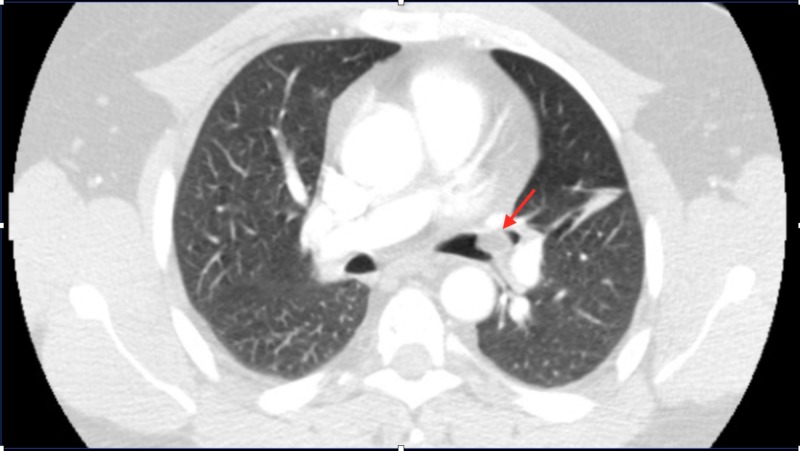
Computed tomography of the chest with IV contrast Endobronchial mass in the left upper lobe (LUL) bronchus (red arrow) resulting in subsegmental atelectasis is noted. IV: intravenous
